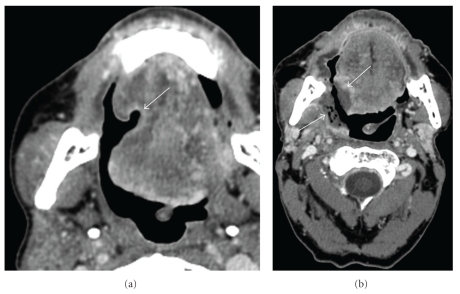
Benign and malignant soft tissue ulceration: (a) Axial contrast-enhanced CT of the oral cavity (soft tissue window) shows a benign ulceration (arrow) without an associated soft tissue mass. (b) Axial contrast-enhanced CT (soft tissue window) shows recurrent tumor characterized by irregular enhancement along the floor of the ulceration (arrows).
